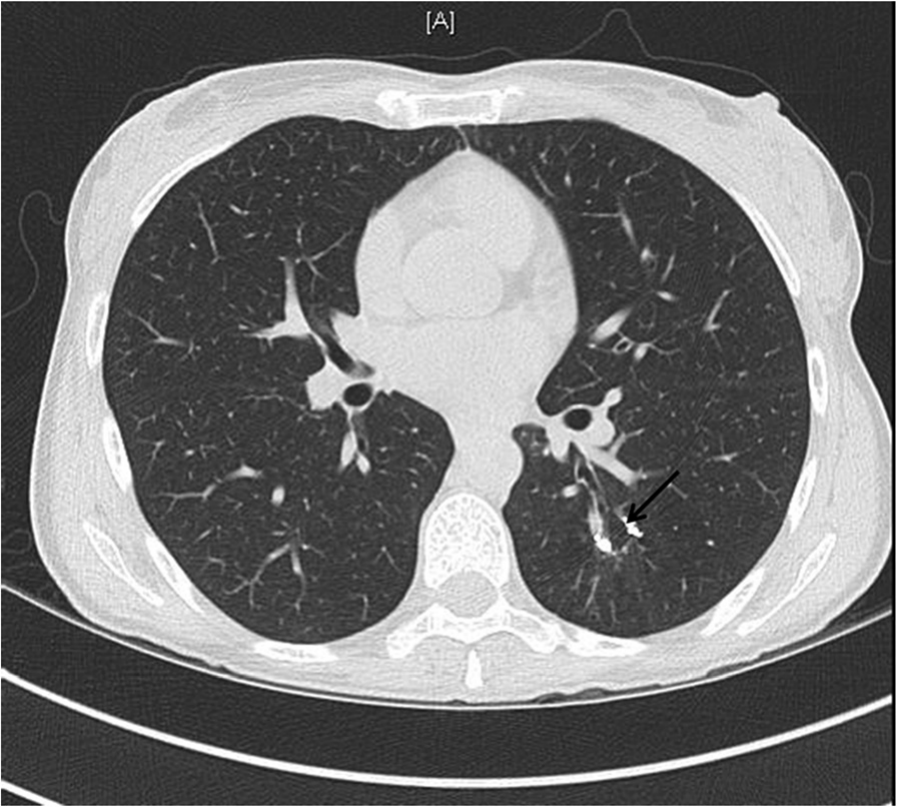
Axial computed tomography image at same level as Figure1performed 4 years after embolization shows metallic coils and obliteration of pulmonary arteriovenous malformation (arrow).
